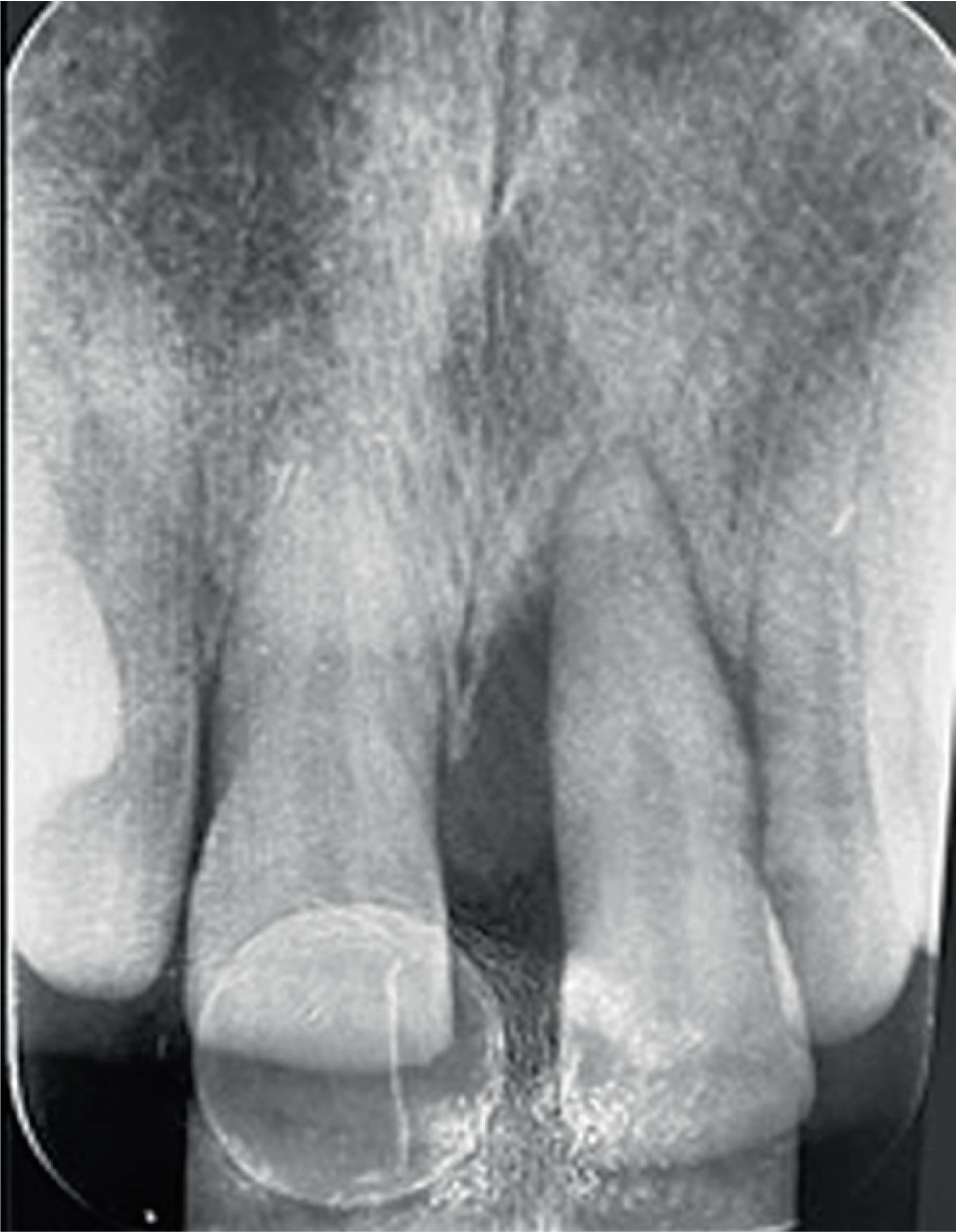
Periapical radiograph showing alveolar bone loss on the mesial surface of the left maxillary central incisor, with slight root displacement but no evidence of internal resorption or periapical changes.
